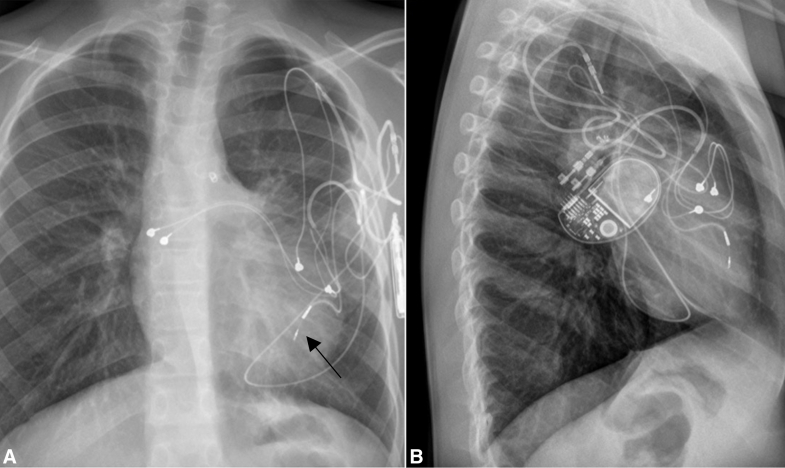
Chest x-ray images after SelectSecure lead implantation. Shown are transmyocardial position of SelectSecure MRI SureScan 3830 bipolar lead stimulating the LV (*black arrow*), conventional epicardial leads on the right atrium, and capped dysfunctional conventional epicardial lead in the left axillary extrathoracic pocket. A, Posterior-anterior view. B, Lateral view.
